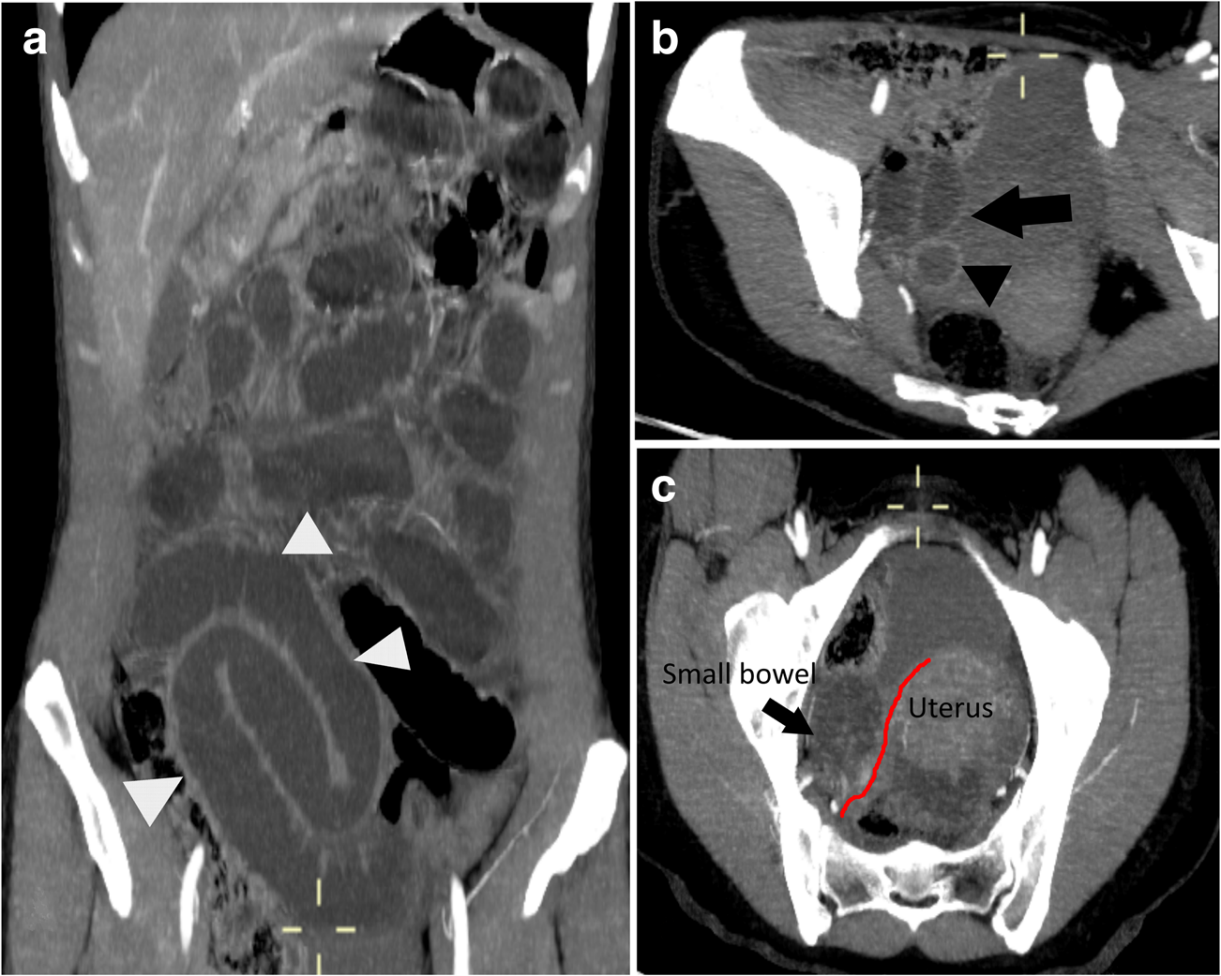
CT scan. (a) Coronal CT scan section showing small-bowel dilatation (white arrowheads). (b) Left oblique CT scan section showing a closed-loop image in the right iliac fossa (black arrow) and herniated loop with thickened walls (black arrowhead). (c) Transverse CT scan section showing thickening of the broad ligament (red mark)
